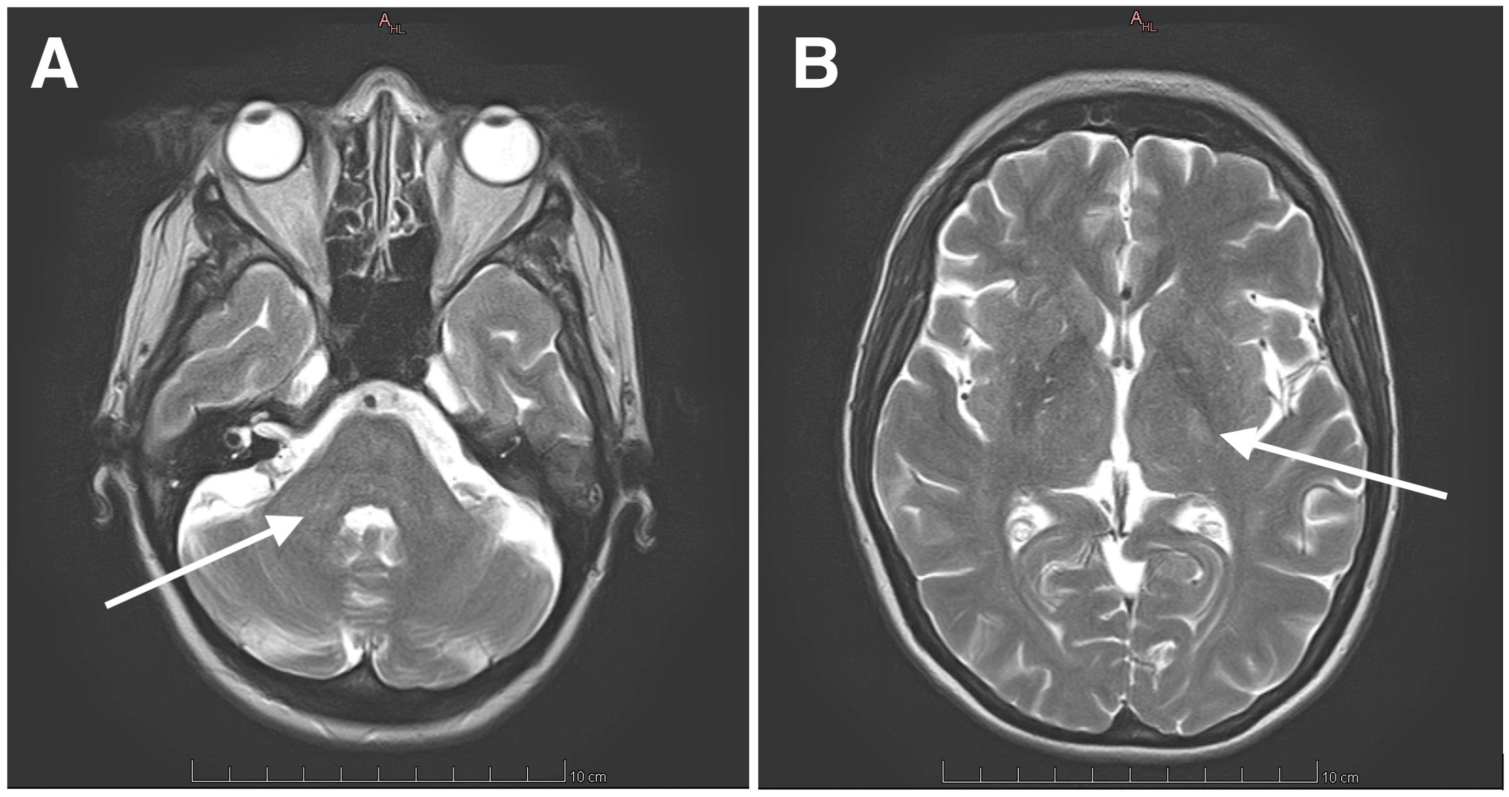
Six-month cerebral MRI with minimal T2-weighted hyperintense lesion on the middle right cerebellar peduncle (arrow, Panel A) and residual lesion on the posterior limb of the left internal capsule (arrow, Panel B) displaying less T2-weighted hyperintensity compared with Figure 1.
